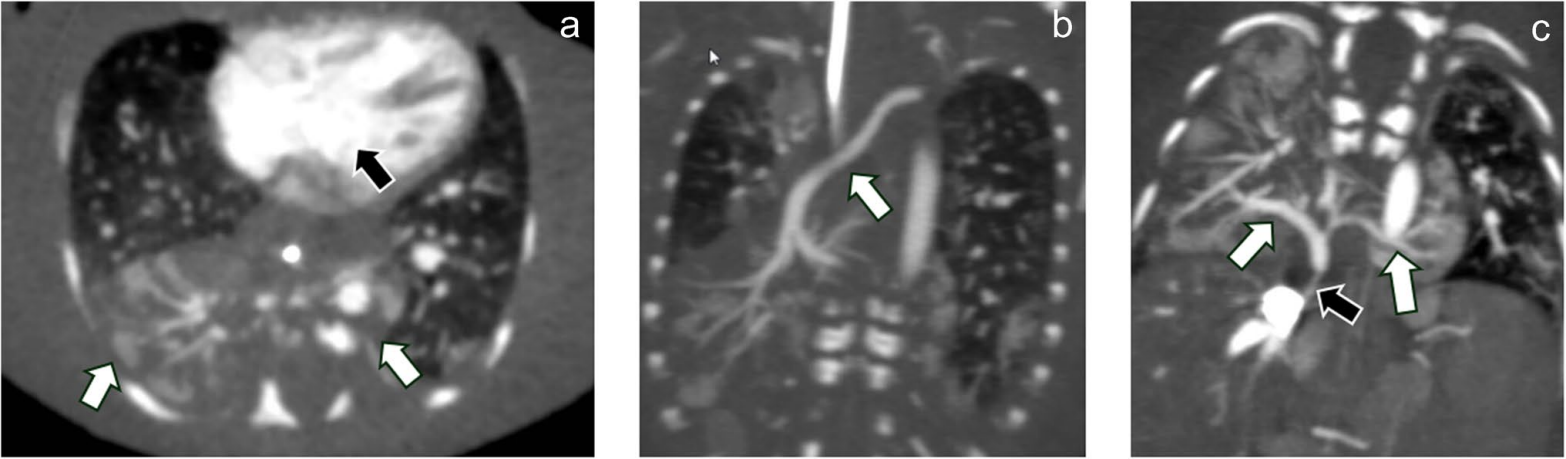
Thoracic malformations. Axial (a) and coronal (b, c) CT angiographic images of the chest demonstrate an extralobar sequestration with a “horseshoe” configuration centered in the right lung base, crossing midline (*white arrows* in a). Note how the blood vessels within the sequestered lung cross midline. A dominant feeding artery (*arrow* in b) originates from the dorsal thoracic aorta near the left subclavian artery origin, taking an oblique supero-inferior mediastinal course to the right base. Draining veins (*white arrows* in c) empty into the inferior vena cava through a narrow channel (*black arrow* in c). There is a ventricular septal defect in this patient with double outlet right ventricle (*black arrow* in a)
